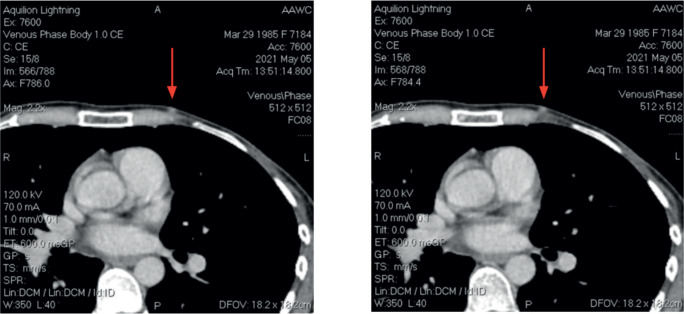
CT Images at third recurrence.
